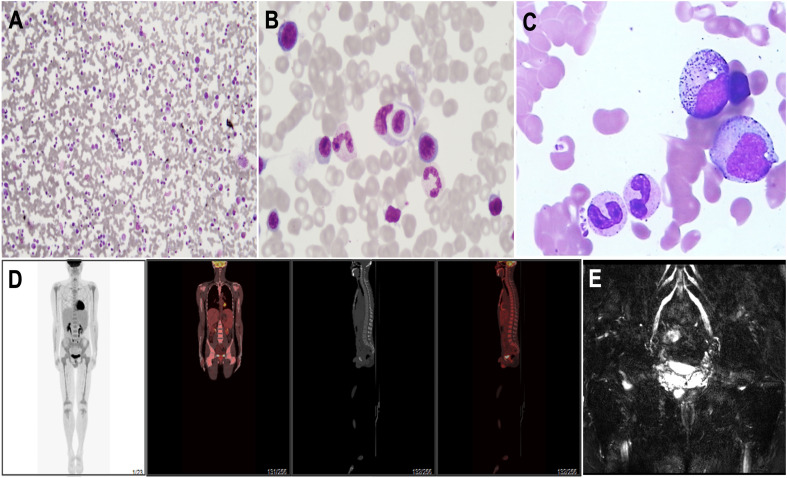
Bone marrow biopsy, PET-CT scan and neuroradiology study of the patients. (A, B) Hyperplastic bone marrow was observed in patient 1, with marked erythroid and megakaryocytic proliferation. The granulocytic lineage is reduced in proportion with partial left shift and some degenerative changes. Occasional atypical lymphocytes and hemophagocytic cells are observed on the smear. (C) In the bone marrow smear of case 2, the lymphocytes exhibited marked morphological irregularity, with some cells showing tail-like projections or pseudopodia. In addition, a small number of phagocytic cells. (D) Fluorodeoxyglucose-positron emission tomography/computed tomography (^18^F-FDG PET-CT) of patient 1 showed hypermetabolic lymphadenopathy and splenic uptake. (E) MRN of the lumbosacral plexus in case 2. MIP of a T2-weighted SPACE 3D sequence demonstrates diffuse enlargement (hypertrophy) and T2w-hyperintense signal of the lumbosacral plexus nerves, indicative of demyelination and inflammation. MIP, Maximum intensity projection; MRN, MR-neurography.
